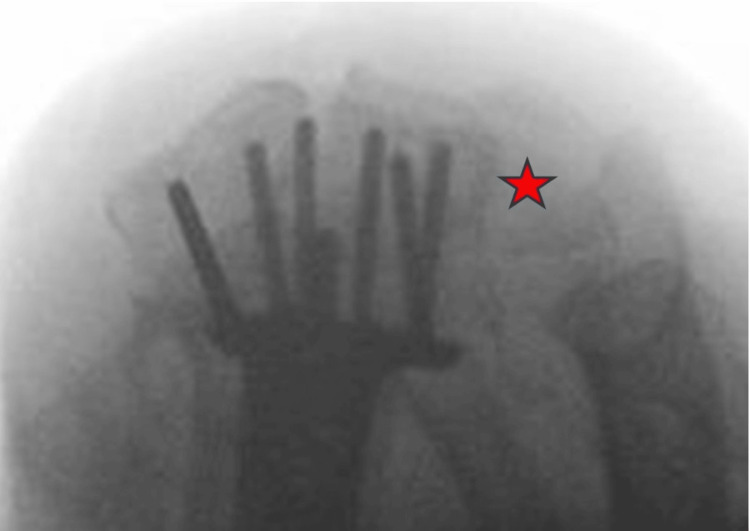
Skyline intraoperative radiograph after volar plate application This shows appropriate length of the distal locking pegs. Also, the sigmoid notch of the distal radius is clearly visible (noted with a red asterisk) and seen to be free from screw penetration.
